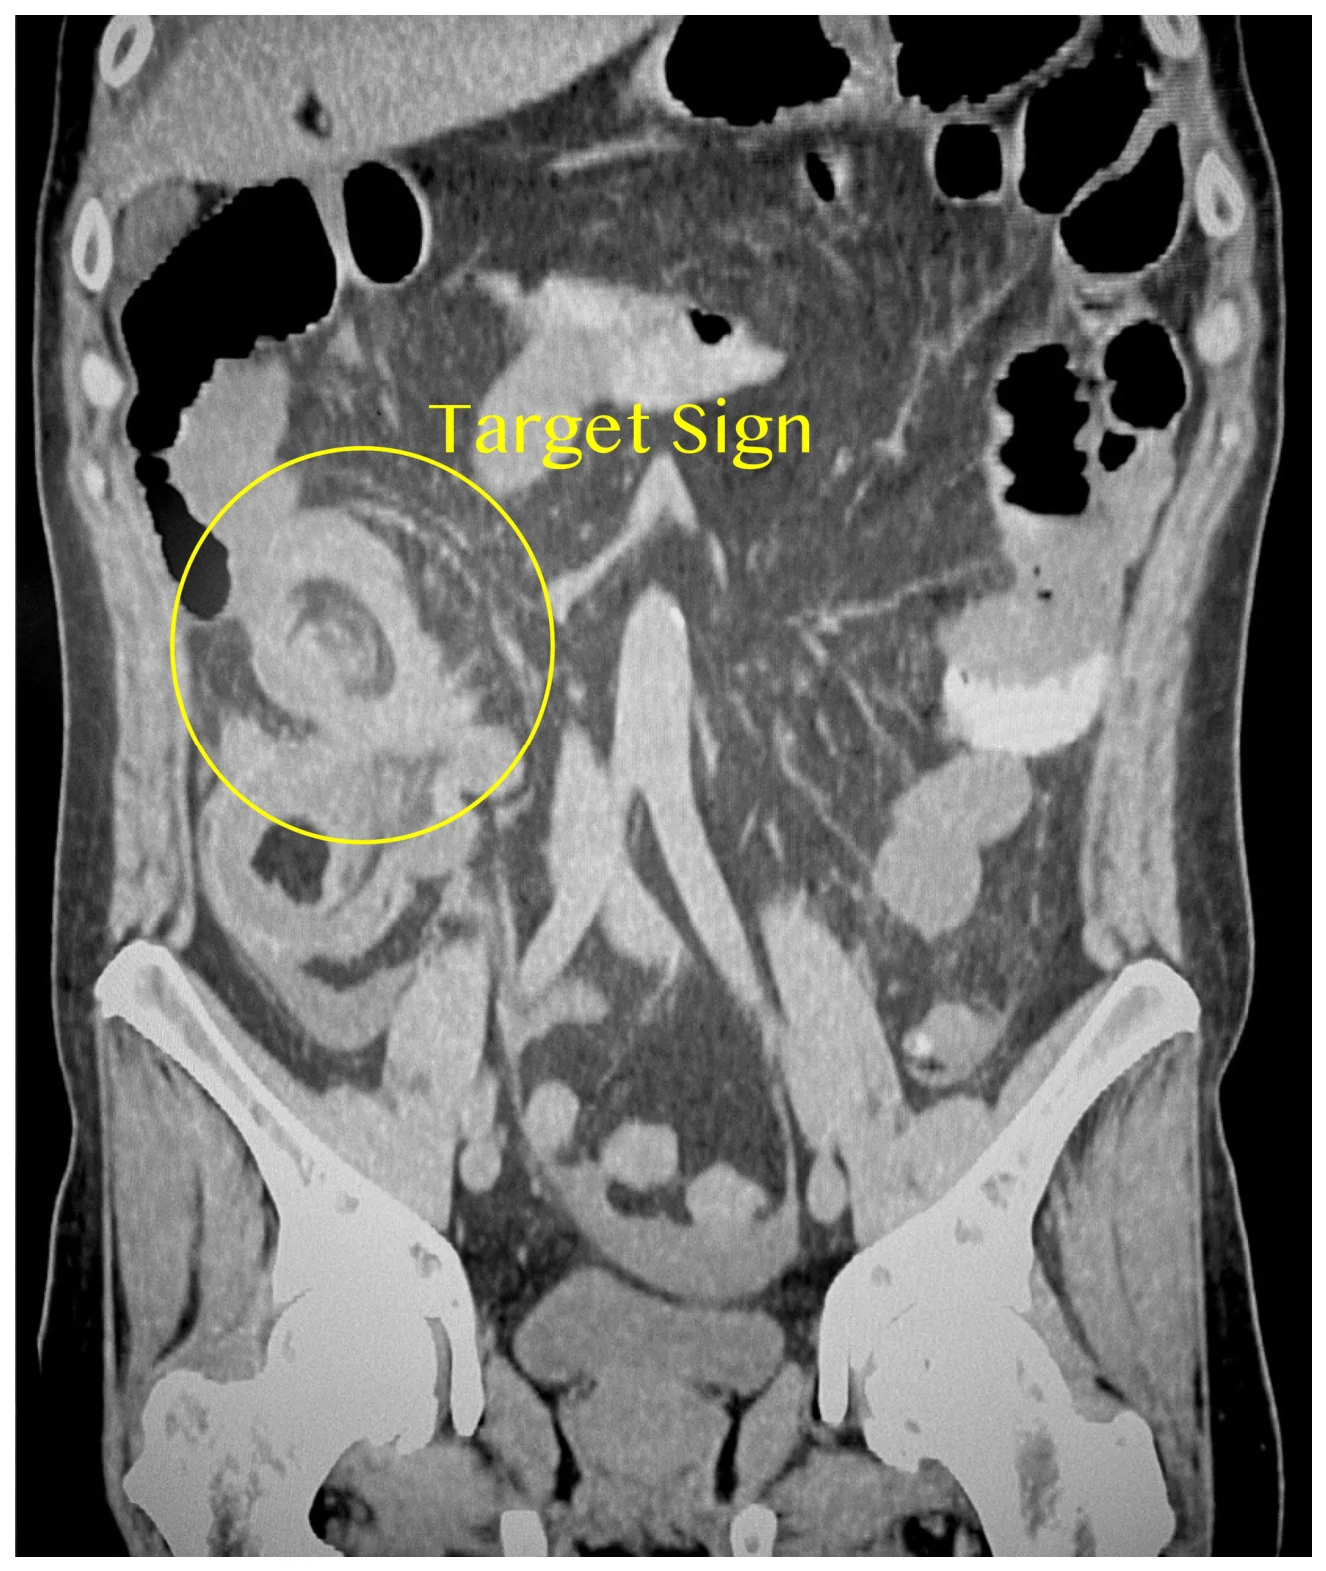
Coronal contrast-enhanced CT image of the abdomen showing ileo–ileo–colic intussusception occupying the ascending colon. The circle identifies the characteristic target configuration, formed by concentric bowel-wall layers with central mesenteric fat and entrapped vessels. The markedly thickened ileal loops demonstrate objective signs of severe ischemic compromise (mural thickening, hypoattenuation, mesenteric fat stranding), without direct demonstration of active contrast extravasation due to the absence of a dedicated arterial-phase acquisition.
